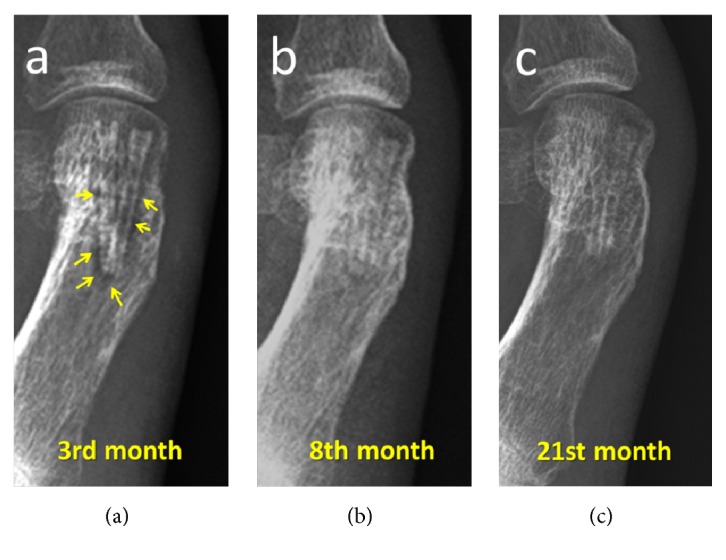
Gradual disappearance of the radiolucent zone around the screw on the 3-month (a) and 8-month (b) follow-up radiographs. (c) Complete disintegration of the Mg screws at 27 months.
